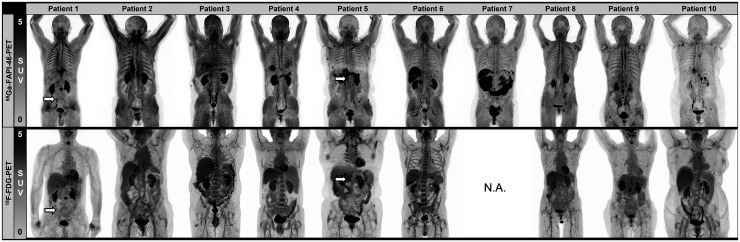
Maximum-intensity projections of ^68^Ga-FAPI-46 and ^18^F-FDG PET for all patients. Tumor lesions that could not be detected by ^18^F-FDG PET are marked with arrows. Tumor sites are listed in Table 2. N.A. = not applicable.
